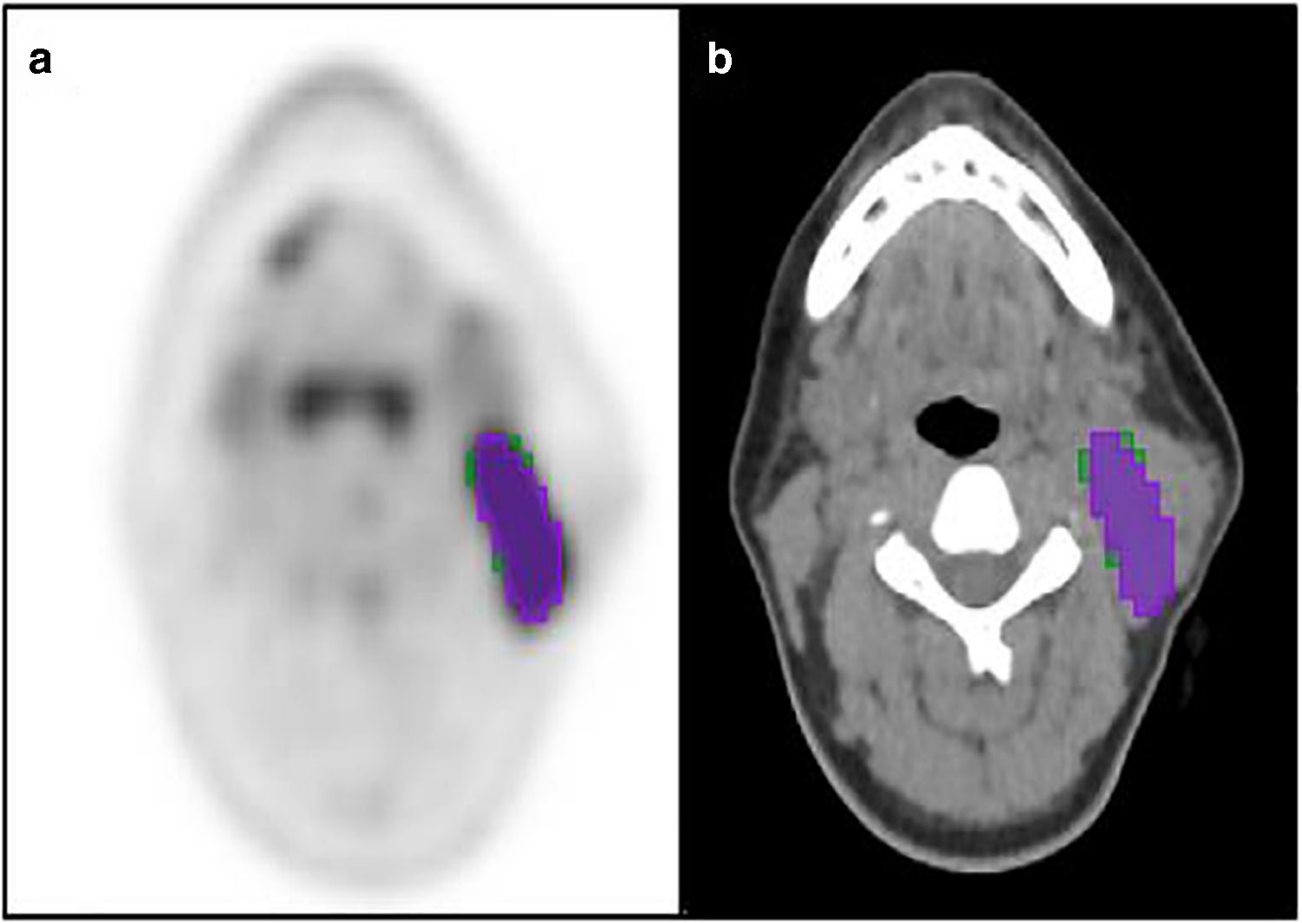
Select axial slice through PET (a) and CT (b) images of a patient with Hodgkin lymphoma demonstrating a pathological left level II lymph node. The purple segmentation represents the original 4.0 SUV fixed threshold segmentation performed using Mirada Medical RTx (v1.8.2) software and the green areas represent the additional area included when segmented with a fixed 4.0 SUV threshold using 3D Slicer (v4.11) software
